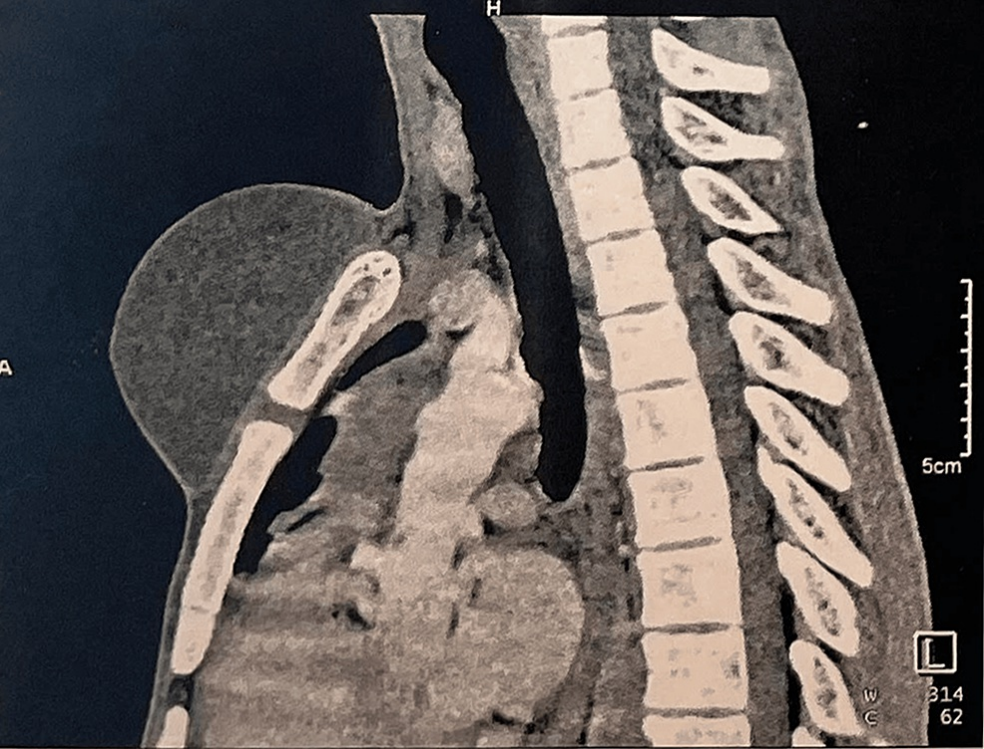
CT scan showing the pre-sternal mass (sagittal section)
